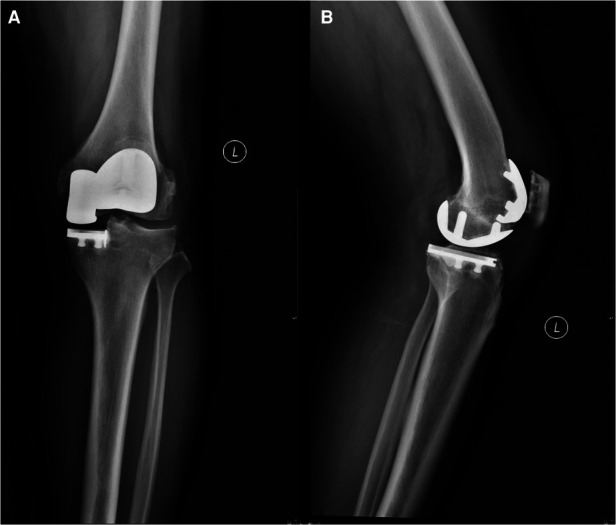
Postoperative knee radiography of patients who underwent BKA. (A) Anteroposterior view; (B) lateral view.
